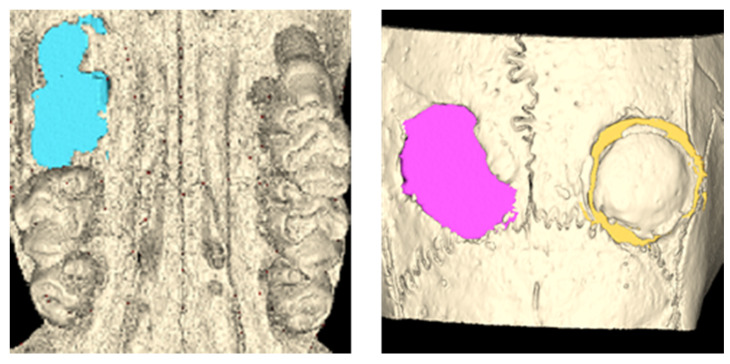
Volumetric measurement using μ-CT. After a ROI was delineated according to the defect, the border of the defects was automatically generated for each slice. The defect volume was calculated through 3D reconstruction.
